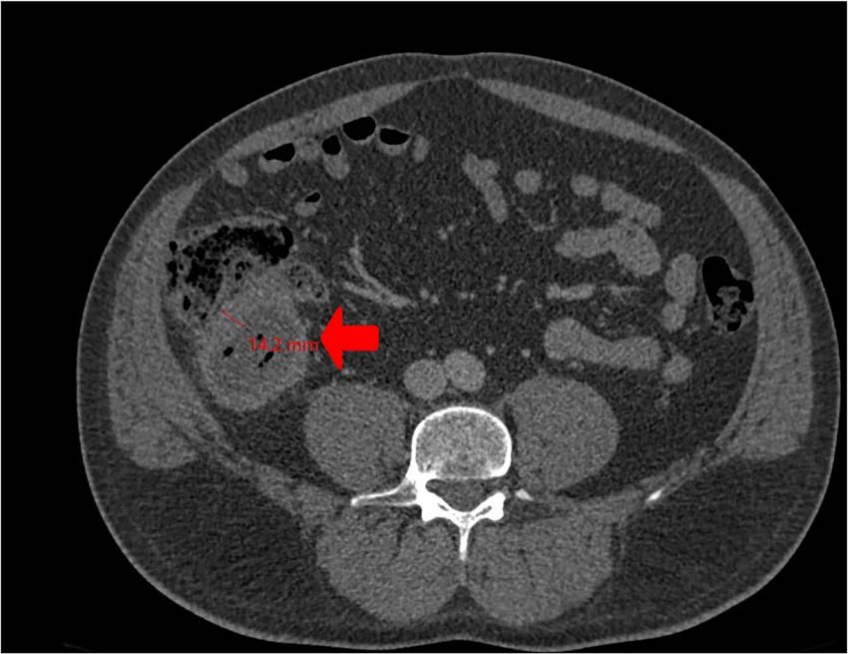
Cross-sectional CT view shows the mass measuring 13 × 5.2 cm with significant wall thickening measuring 14.2 mm and fat stranding. The contents are a mixture of fluid and gas, consistent with appendicitis.
